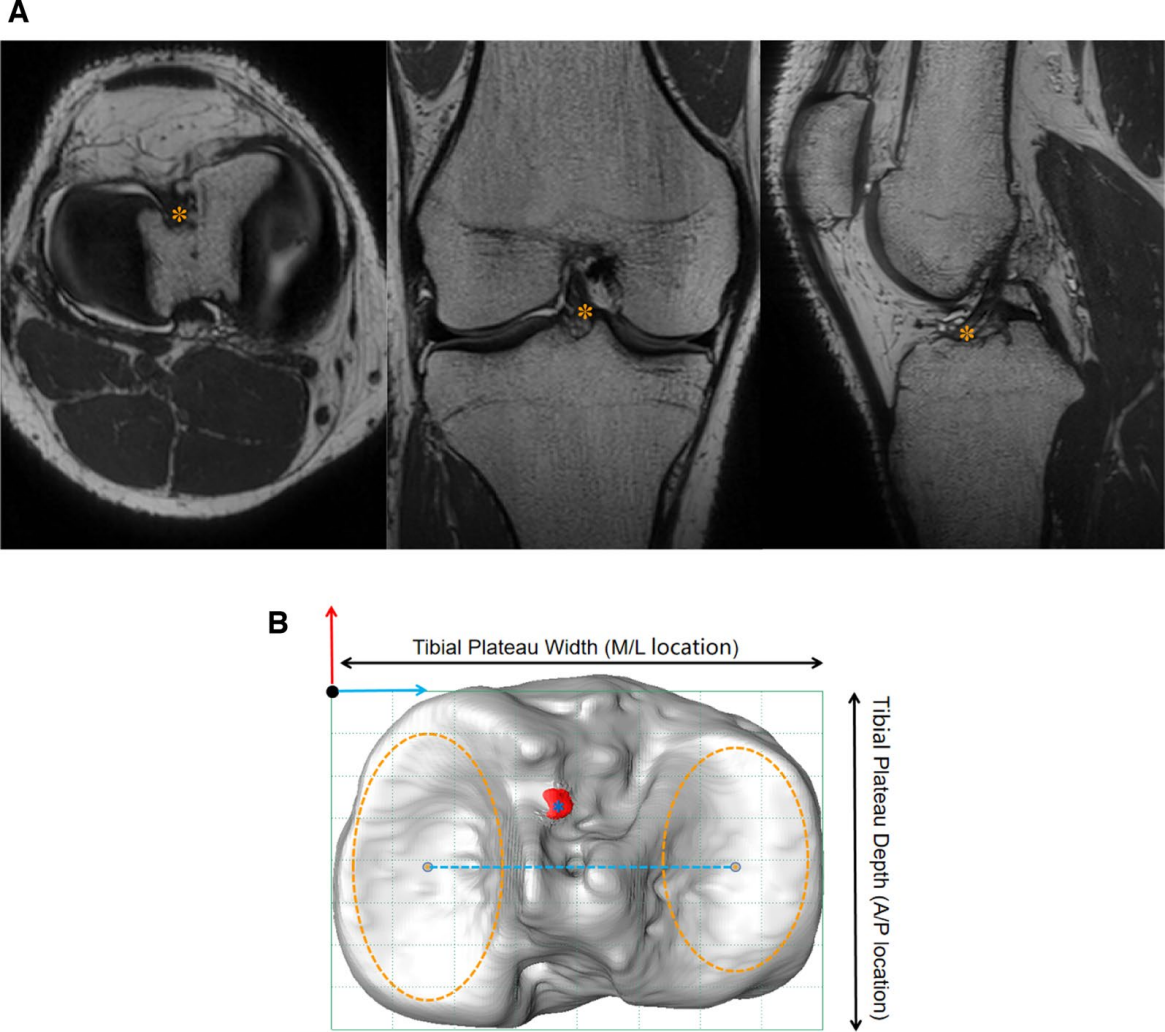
a 3D MRI clearly showing the ACL in three orientations. The ACL tibial footprint location is marked with an* orange star*. b Three-dimensional surface models of the right tibia and the tibial footprint area were reconstructed. The anatomical coordinate system of the tibia (tACS) was also created. The origin is shown with a* black dot*, and the medial/lateral axis connecting the centers of the best-fitted ellipses on the articular surfaces of the medial and lateral tibial plateaus is shown with a* blue line*. The anterior/posterior axis is shown with a* red arrow*. Within the best-fitted plane, a bounding box was defined by the depth and width of the tibial plateau. The origin of the tACS was then moved to the most anterior and medial point of the bounding box
